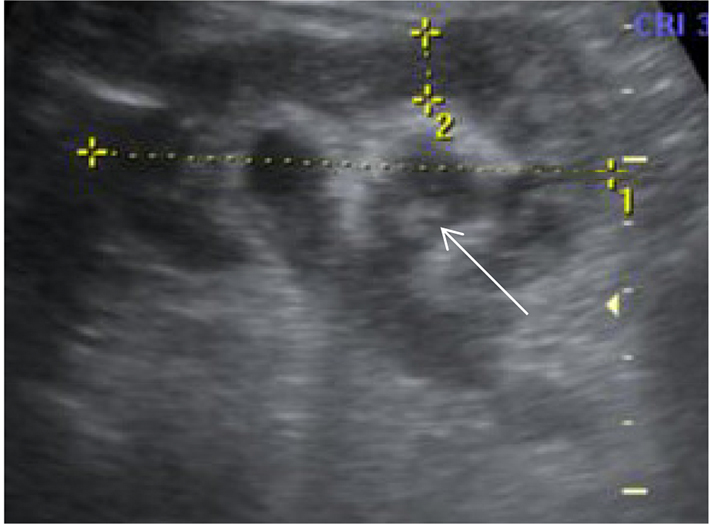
Ultrasound image showing hydronephrosis with a dilated pelvicalyceal system. Hyperechoic material is noted within the interpolar calyx (long white arrow).
